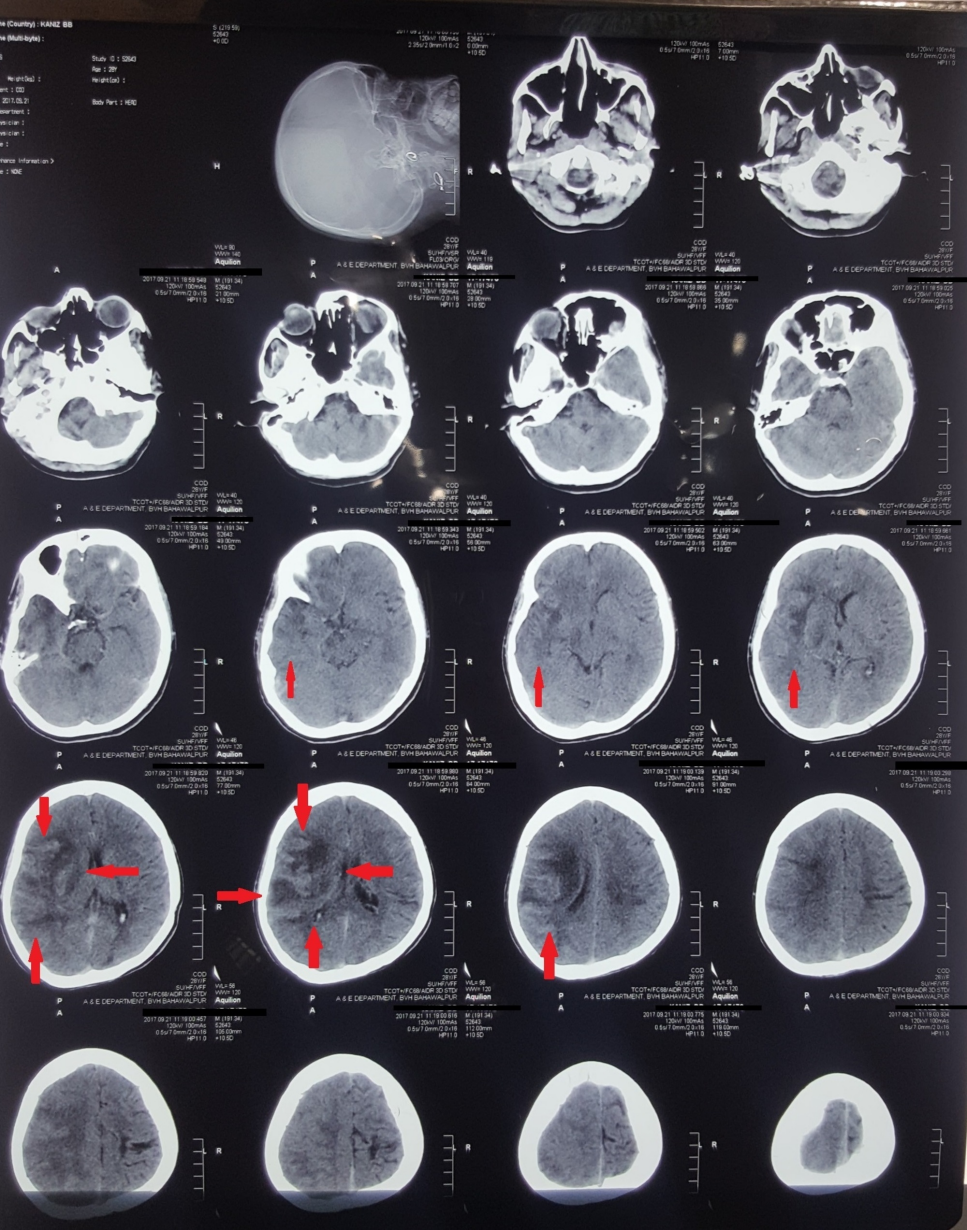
Computed tomography (CT) scan showing changes in the right temporoparietal region
